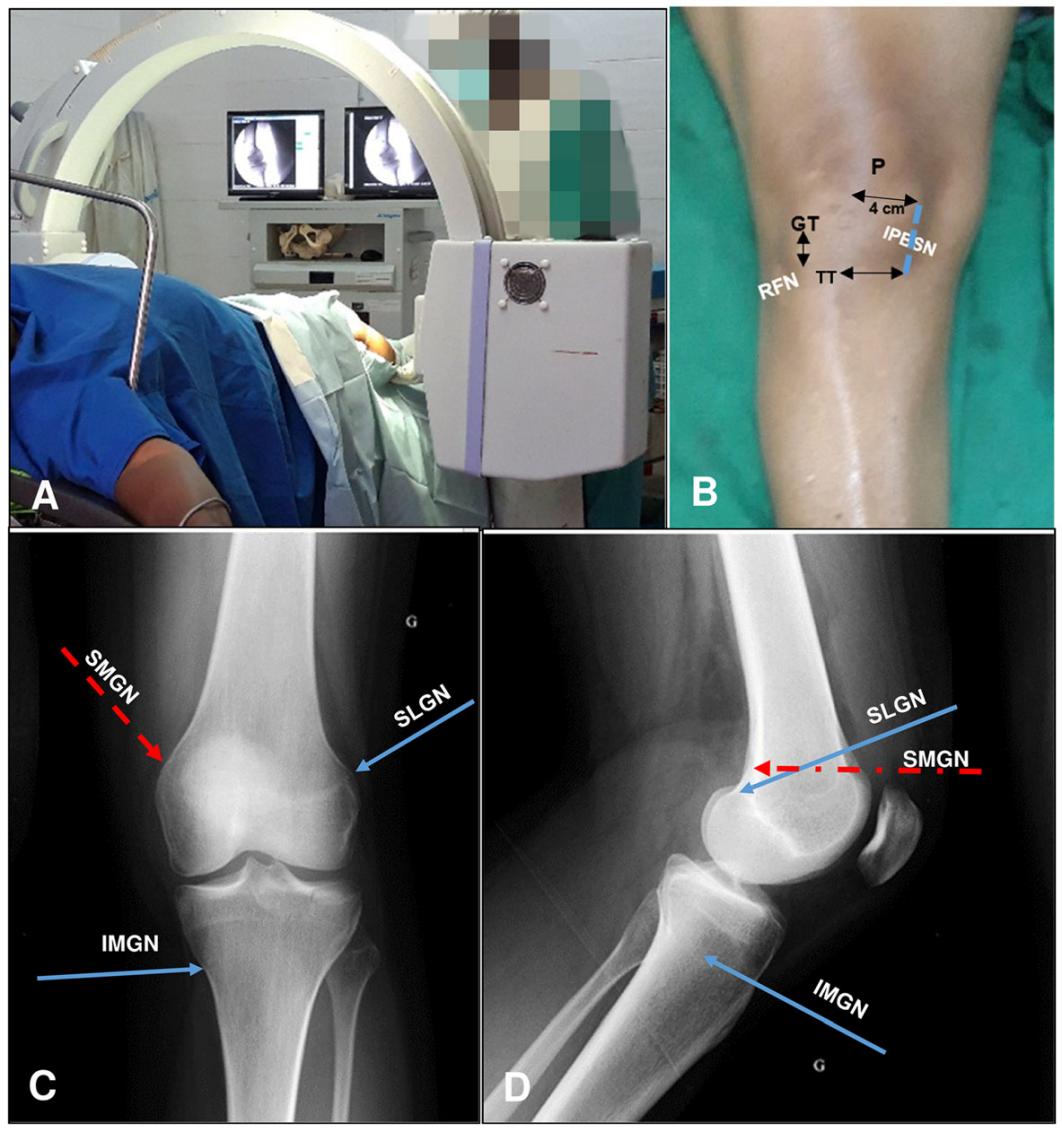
Anatomical targets for fluoroscopic guided genicular nerve blockade. a Installation b Landmarks for infrapatellar branch of the saphenous nerve (Dashed blue line represents the treatment line) and recurrent fibular nerve (blue point) targeting. c Anterior-Posterior X-ray view of the knee. Landmarks of Cannula placement for targeting the Superior medial genicular nerve (Dashed red arrow), superior lateral genicular nerve (Upper blue arrow) and inferior medial genicular nerve (lower blue arrow). d Landmarks for targeting the same three nerves on the lateral view of the knee. SLGN, superior lateral genicular nerve; SMGN, superior medial genicular nerve; IMGN, inferior medial genicular nerve; IPBSN, infrapatellar branch of saphenous nerve; RFN, recurrent fibular nerve; P, patella; TT, tibial tuberosity; GT Gerdy’s tubercle
